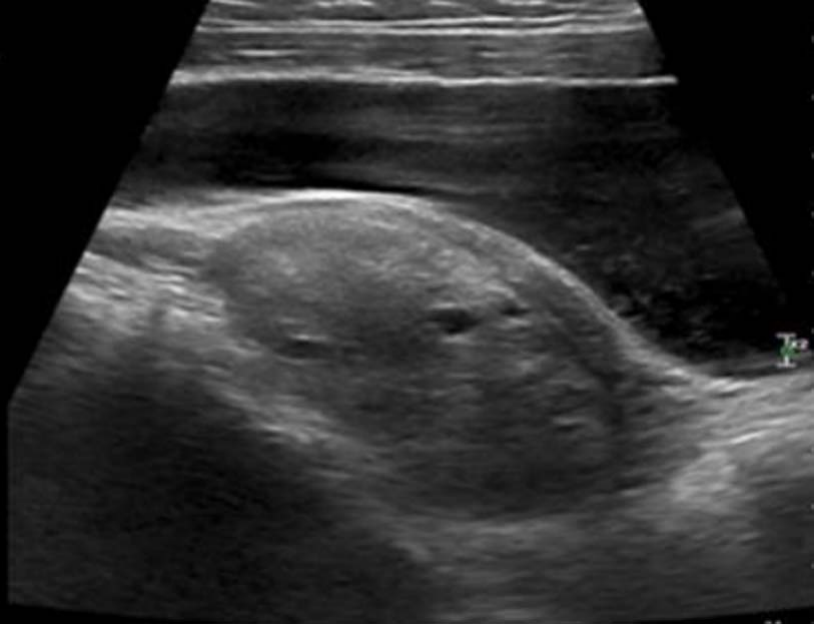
Per abdominal ultrasound image shows a heterogeneous lesion in the left adnexa with some cystic areas.
